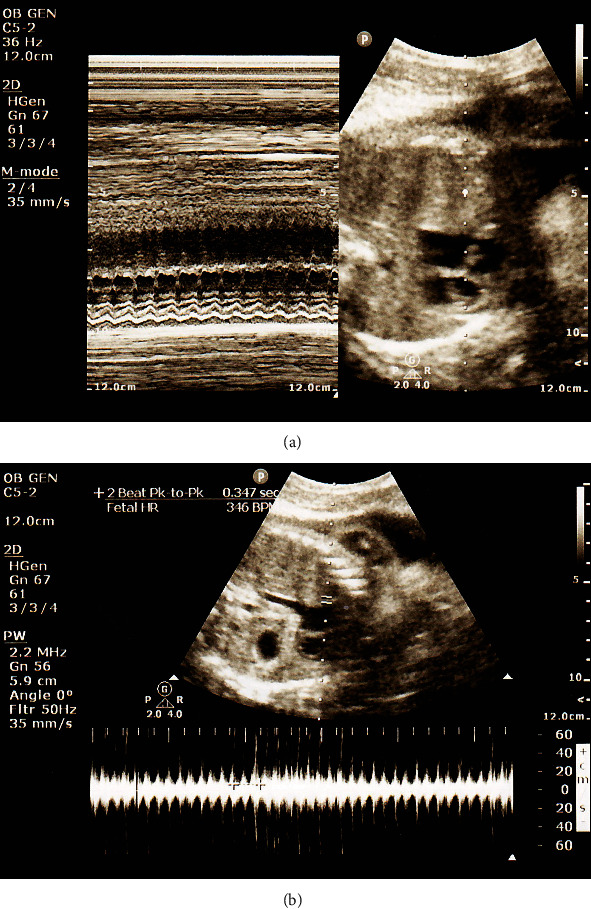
Bedside ultrasound on day of admission, demonstrating fetal tachycardia on (a) M-mode and (b) spectral Doppler mode.
